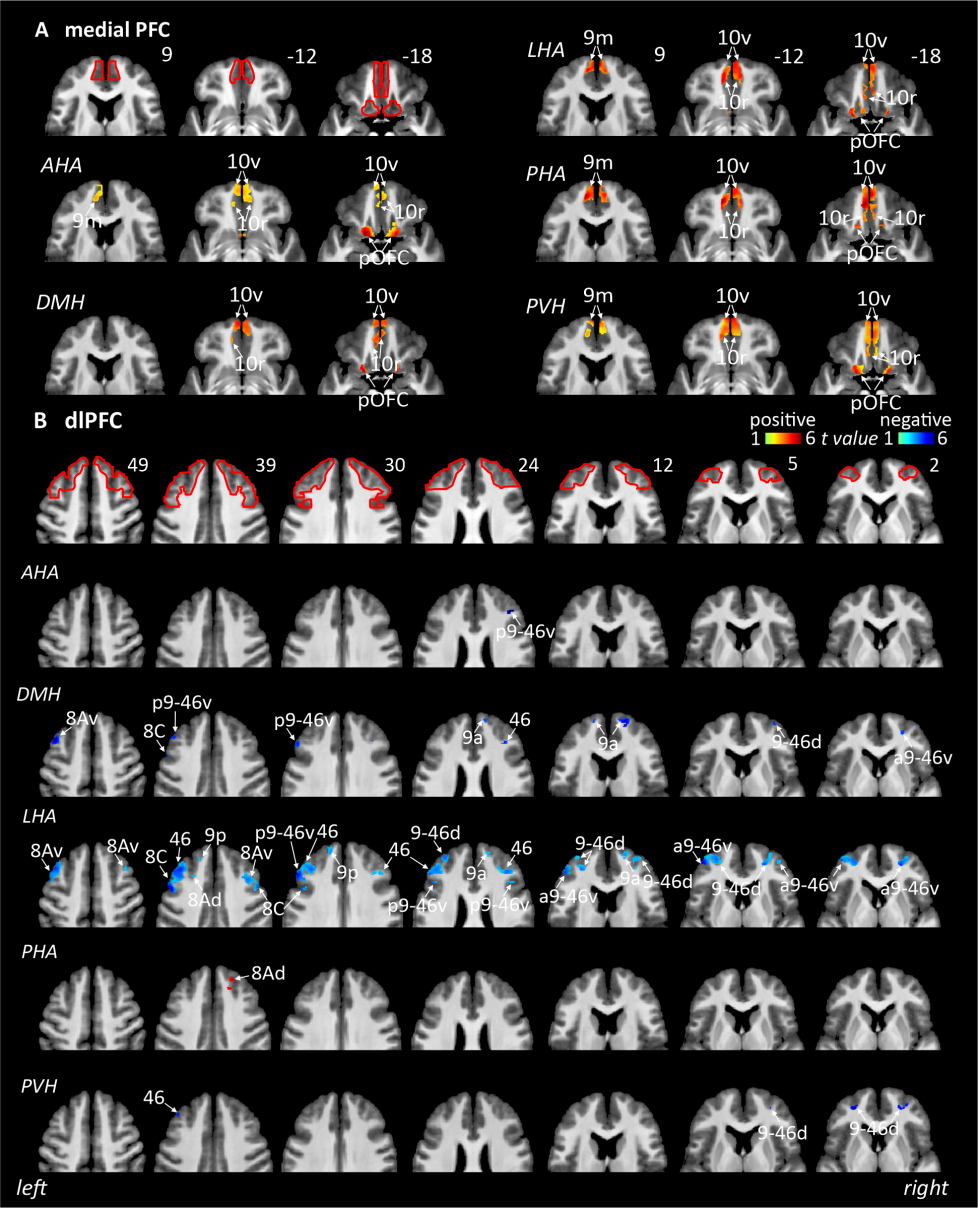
Resting connectivity between six hypothalamic seed regions and the medial prefrontal and dorsolateral prefrontal cortical regions (n = 150). Areas of significant positive (hot colour scale) and negative (cool colour scale) connectivity overlaid onto a series of axial mean T1-weighted anatomical images. Slice locations in Montreal Neurological Institute Space are indicated at the top right of each slice. Red outlines indicating region of interest masked applied for the analysis. (A) Medial prefrontal cortex (PFC). (B) dorsolateral PFC (dlPFC). For abbreviations, see table.
